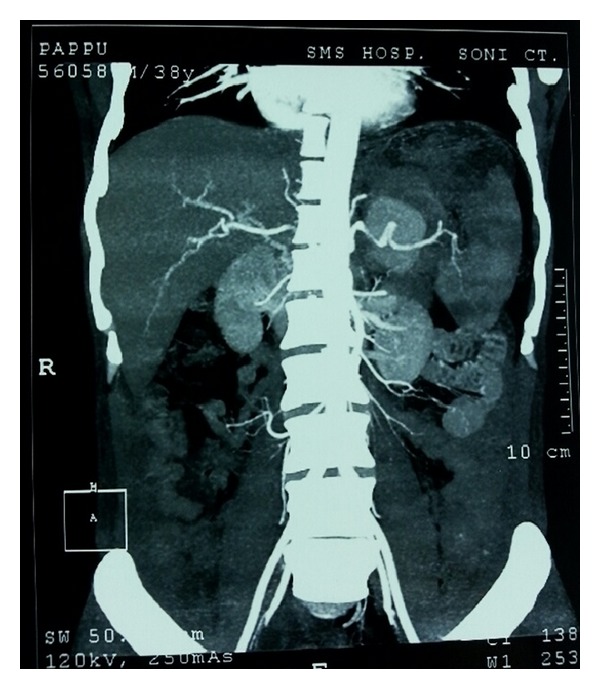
Triple phase CT finally giving the diagnosis of pseudoaneurysm.
